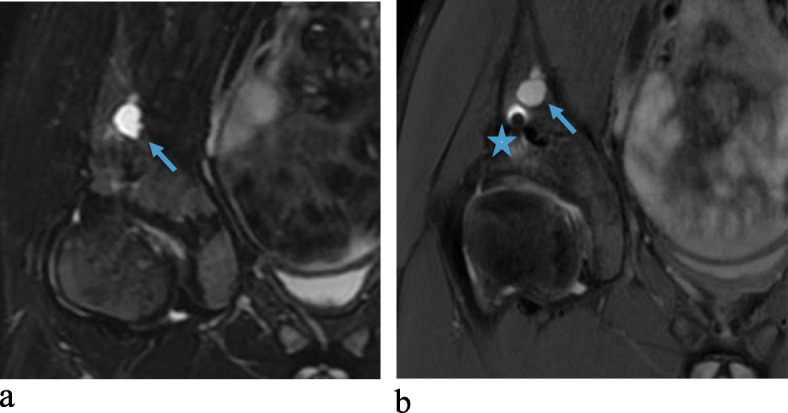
a, b MRI (coronarPD FS/3T) on a 12-year-old boy performed 1.7 and 4.2 years postoperatively, respectively, after a triple osteotomy due to Perthe’s disease. Note the largest bone cyst (arrow, 10 mm) observed in the study. In Fig. 2b, MRI (coronar T2W FS/1.5T) note the metal artefact (star) and the remaining bone cyst (arrow, 12 mm). The screws are completely resorbed and replaced by bone
